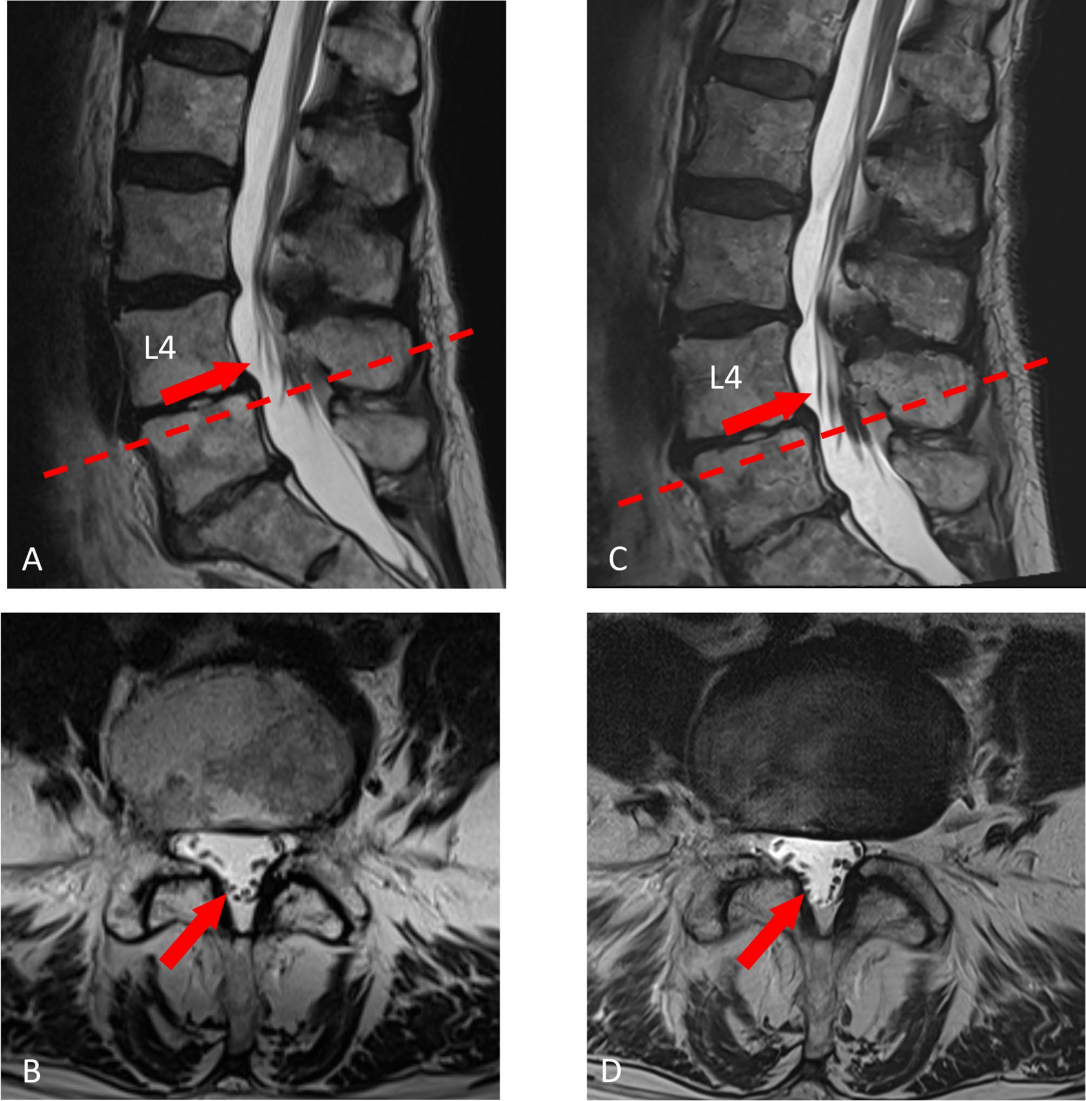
MRI of the lumbar spine of Case 2 one day (A, B) and two months (C, D) after the epidural steroid injection with sagittal (A, C) and transverse (C, D) T2-weighted sections. Both MRI show moderate degenerations of the lumbar spine but a normal appearance of the conus medullaris and cauda nerve roots and no signs of arachnoiditis (arrows in A – D). Note: the dashed line in A describes the orientation of the transverse section B at level L4/5, the dashed line in C describes the orientation of the transverse section D at the level L4/5
